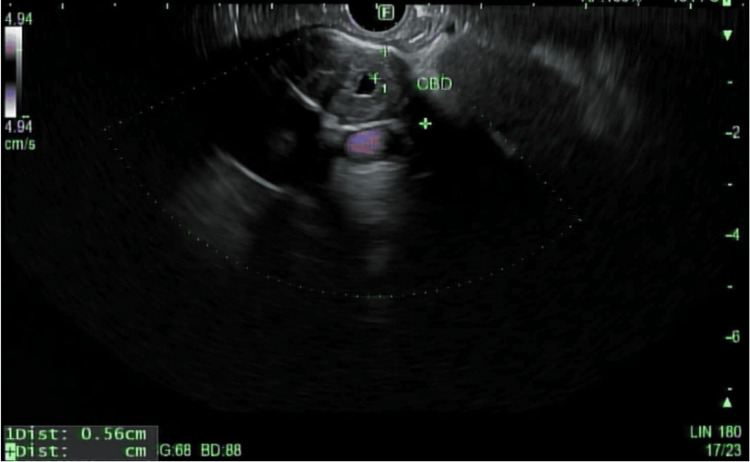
Endoscopic ultrasound demonstrating diffuse thickening of the common bile duct (CBD).
